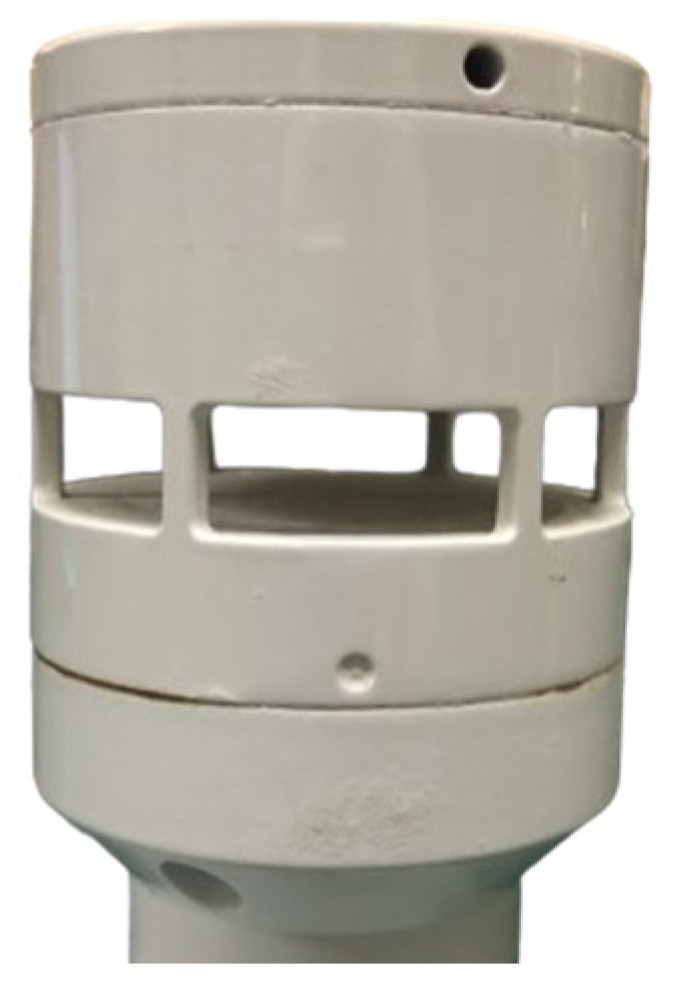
Ultrasonic resonance wind speed and direction sensor.
